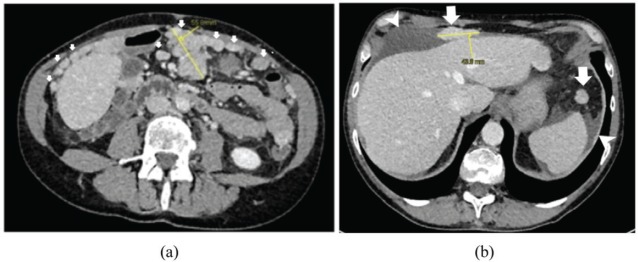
Axial contrast-enhanced CT images show multiple omental nodules [white arrows in (a) and in (b)], some of which are measured in mm [yellow callipers in (a) and in (b)] and peri-hepatic and peri-splenic ascites [arrowheads in (b)].
